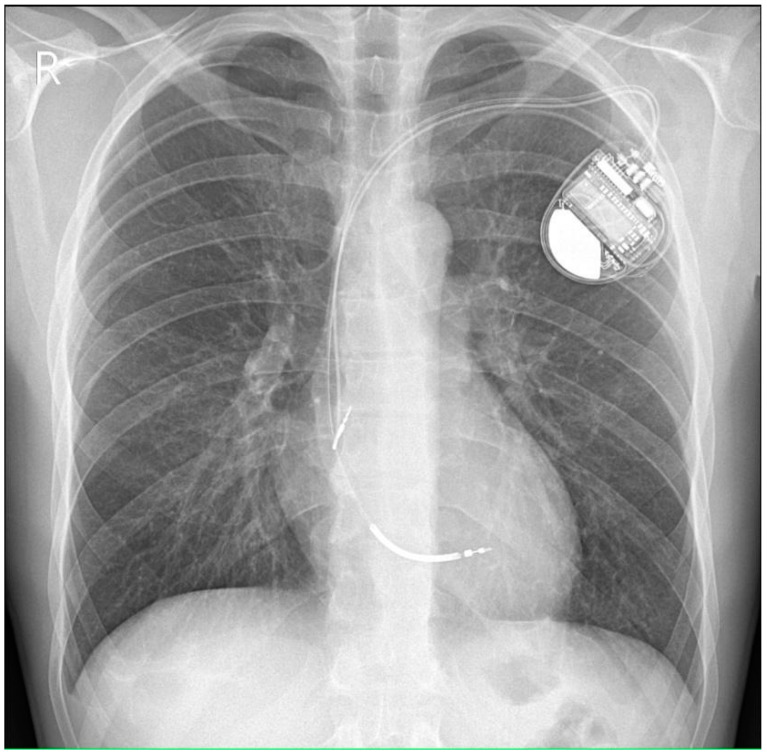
Chest X-ray in the PA projection—condition after implantation of a dual-chamber cardioverter-defibrillator—a single-coil defibrillating electrode with a tip in the middle of the interventricular septum and atrial electrode with a tip in the right atrium appendage. No other abnormalities are seen in the X-ray image.
